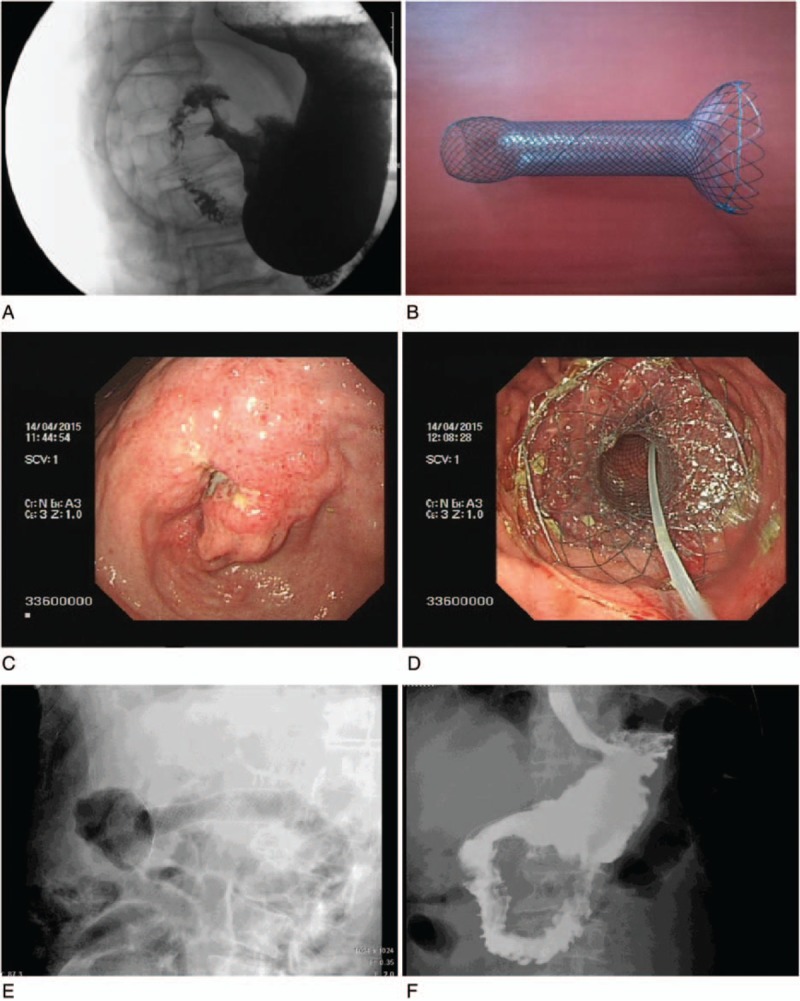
Use of a partially covered cup stent in treatment of cup shaped GOO. A, Distal gastric outlet cup shaped obstruction. B, Cup stent. C, Endoscopic view of a tumor in the distal gastric cavity. D, Endoscopic view of the proximal cup stent at the pyloric area. E, Confirmation of stent deployment by fluoroscopy. D, Barium contrast radiography shows contrast agent filling the stent. GOO = gastric outlet obstruction.
